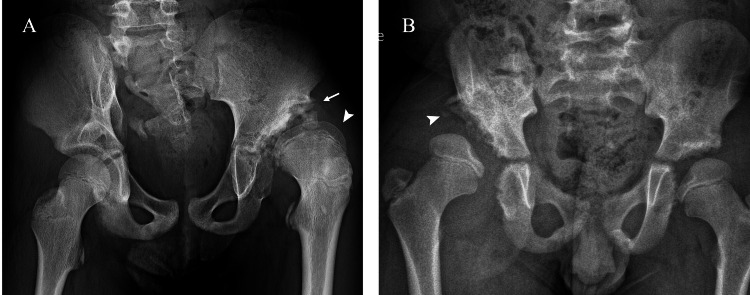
Neuropathic arthropathy (NA) of the left hip in a boy under treatment for lipomyelomeningocele at birth (A). Anteroposterior (AP) pelvic radiograph at 10 years of age (A) shows flattening of the femoral head and thickening of the femoral neck (arrowhead), superior subluxation and the formation of a neo-acetabulum (arrow) due to chronic dislocation. Dislocation of the right hip without underlying NA in an 8-year-old boy with hereditary sensory and autonomic neuropathy type IV (B). AP radiograph (B) shows lytic changes of the iliac bone (arrowhead) without anomalies of the femoral head
